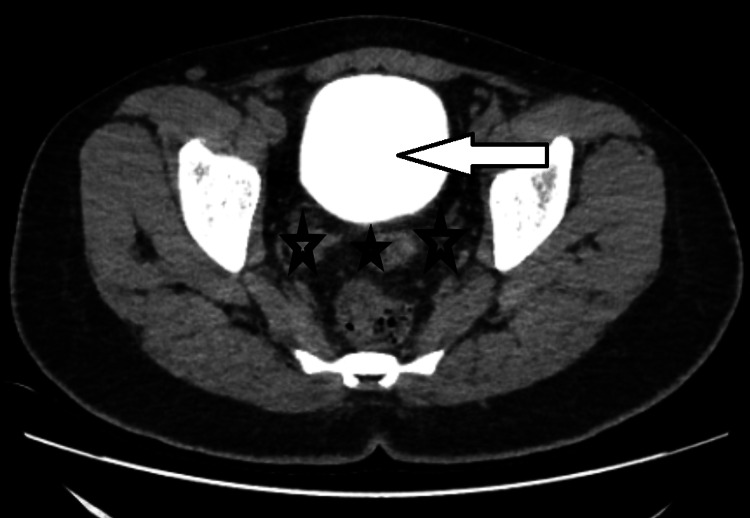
CT cystogram axial view on day 10 after surgery (supine position) showing contrast in the bladder (white arrow) with no contrast leak in the peri-vesical area (black stars). White arrow: urinary bladder with contrast, black stars: peri-vesical area with no contrast leak CT: computerized tomography
